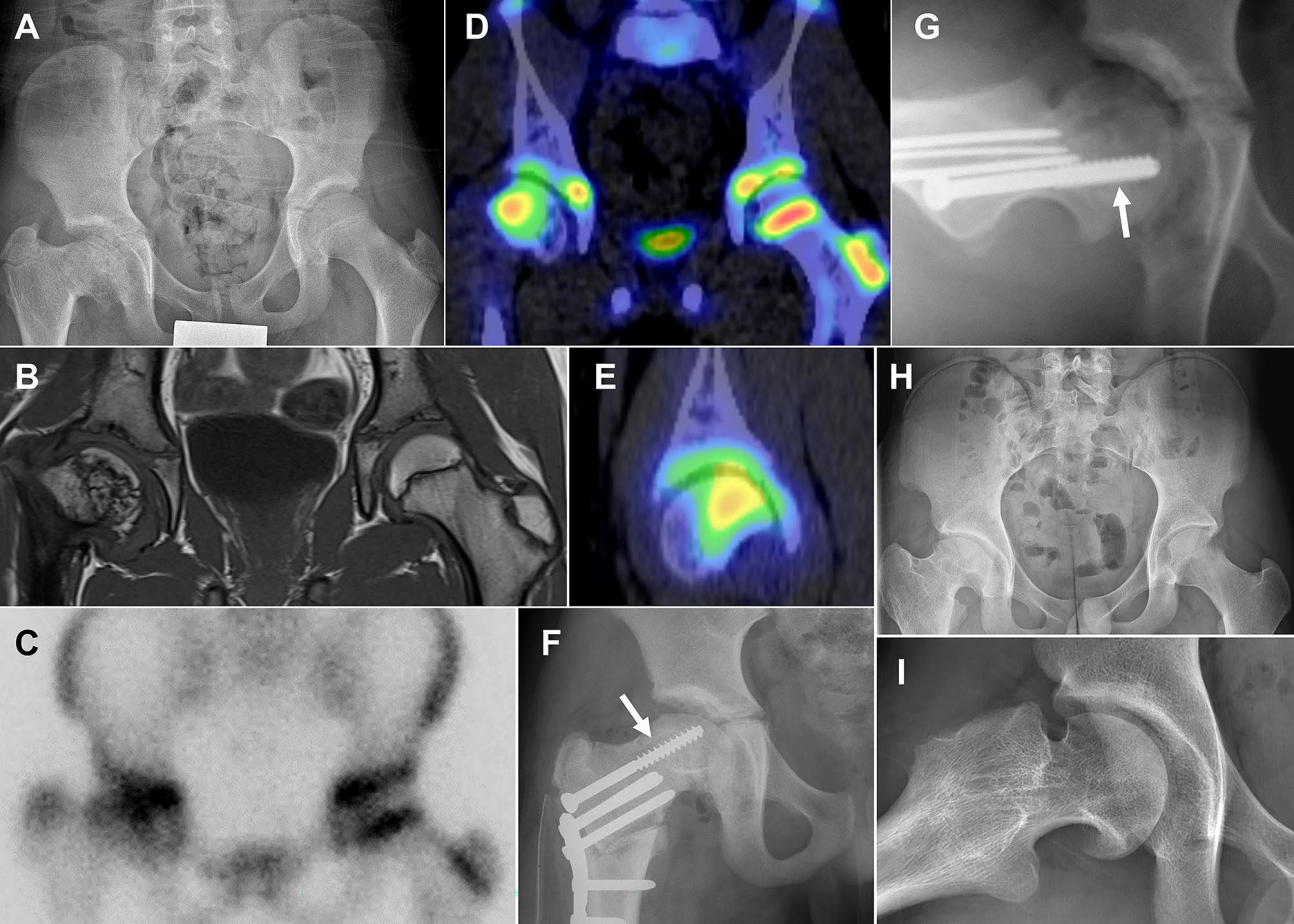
Advantage of bone SPECT/CT for evaluating growth disturbance of the proximal femur. A standing anteroposterior hip radiograph of a 10-year-old boy shows right coxa vara recurrence following proximal femoral valgus osteotomy due to septic hip sequelae (A). On T1-weigthed MRI, the physis exhibits irregular-contour, but a bone bridge is not definitely identified. (B). The proximal femoral physis is inseparable from the acetabular cartilage on planar scintigraphy derived from SPECT/CT (C) but is distinguishable on fused SPECT/CT (D and E). On bone SPECT/CT, the uptake of ^99m^Tc-DPD is evident only at the posterolateral (D and E) aspects in the right proximal femoral physis, which is considered the cause of recurrent deformity. A step-cut proximal femoral valgus osteotomy was performed along with the insertion of a transphyseal screw (arrows) in the posterolateral physis to prevent recurrence of coxa vara (F and G). No recurrence was noted until skeletal maturity (H and I)
